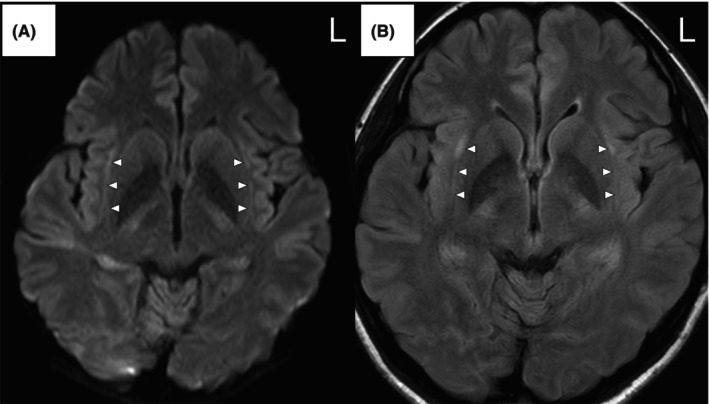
Brain MRI revealing high signal intensity on diffusion‐weighted (A) and fluid‐attenuated inversion recovery (B) imaging within the bilateral claustrum (arrowheads).
